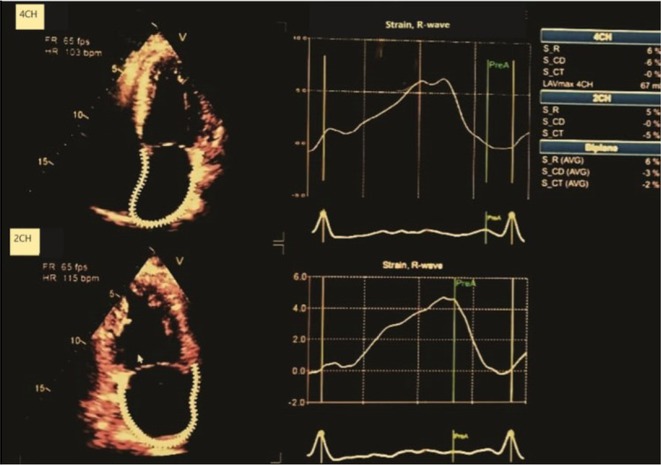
FIGURE 3 Strain curves from left atrial speckle‐tracking, in apical four‐chamber and two‐chamber.
